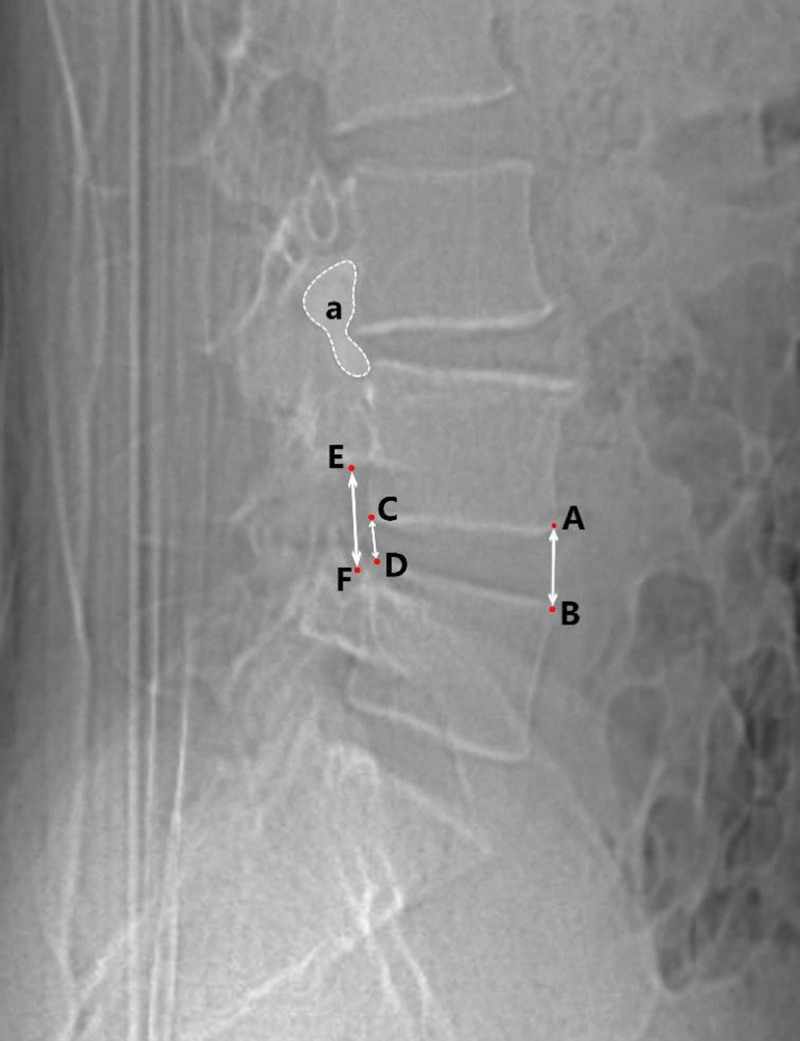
Lateral X-ray of lumbar vertebra. AB-height: anterior disc height. CD-height: posterior disc height. EF-height: foraminal height. a-area: foraminal area.
